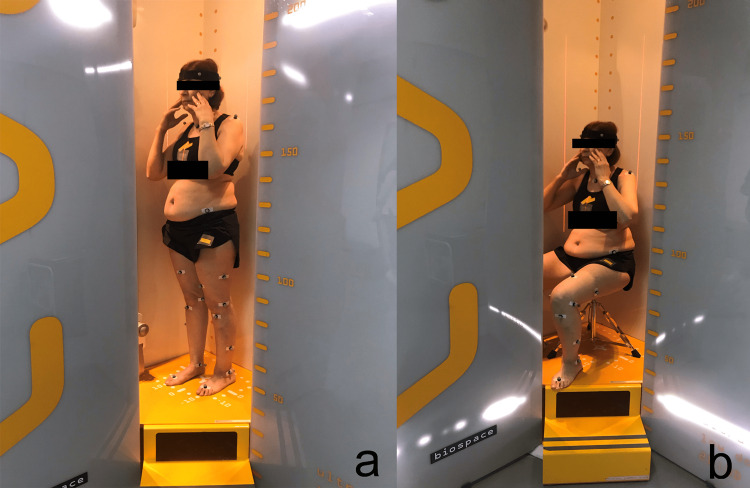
Low-dose biplanar radiograph acquisitions: standing (a) and sitting (b) positions. Authors’ own creations
